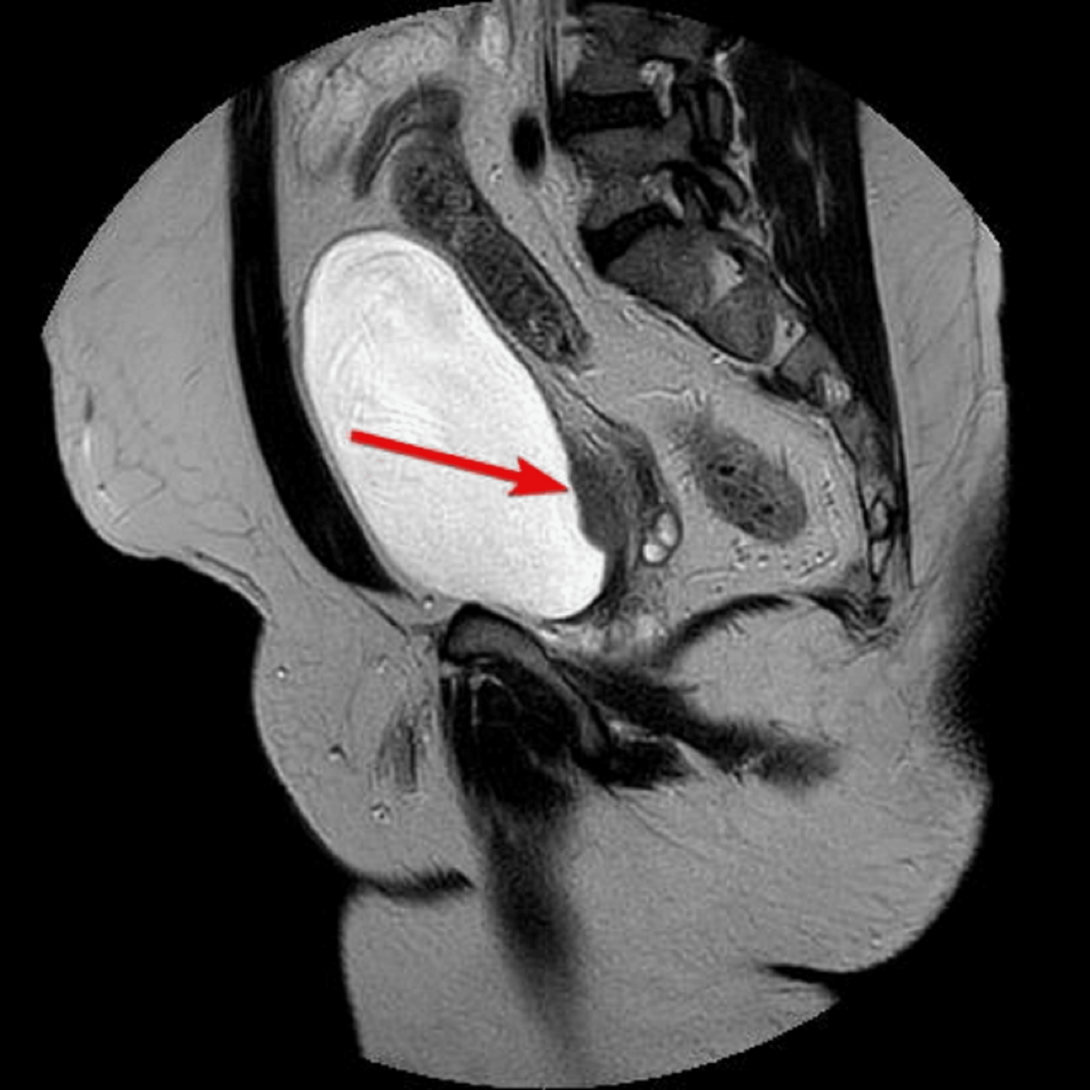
Lesion along the right bladder base visualized on MRI T2 sagittal images.
